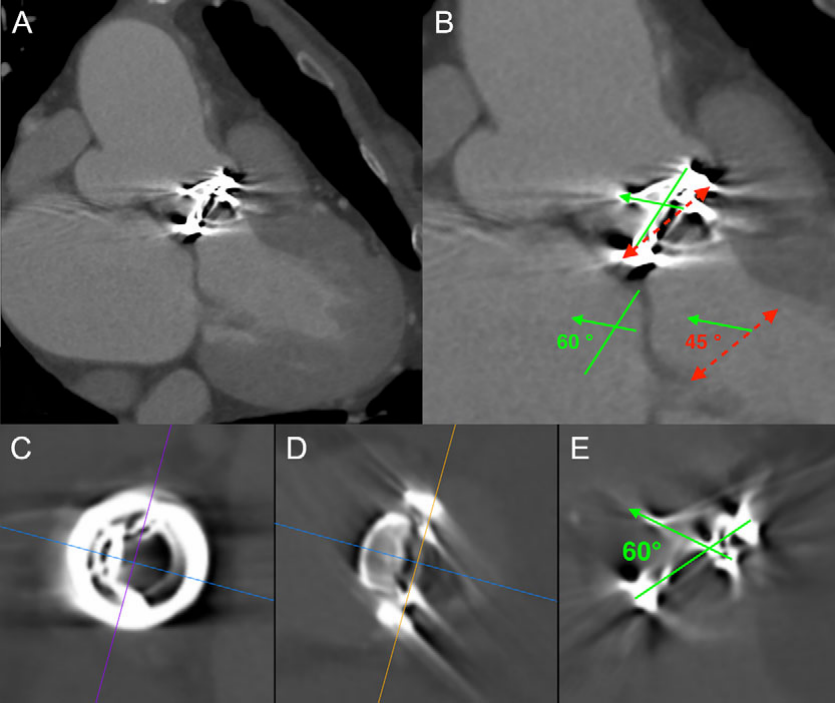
(A) Cardiac computed tomography (CT) angiography revealed a saccular ascending aortic aneurysm (maximum transaortic diameter: 51 mm) arising from the posterior ascending aortic wall just above the sino-tubular junction. (B) Cardiac CT angiography (systolic images) confirmed that the Björk-Shiley valve was tilted by the elevation of the prosthesis in the right-coronary sinus. Red dashed line: Aortic annulus (Basal ring), Green solid line: Prosthetic valve ring, Green solid arrow: Prosthetic valve leaflet. Physiologic opening angle calculated between the leaflet of the prosthetic aortic valve and the aortic annular plane was 45 degrees. (C, D, and E) Prosthetic heart valve assessment with cardiac CT (systolic images). Short axis view (C), frontal view (D), and lateral view (E) of the Björk-Shiley spherical-disc valve in the aortic position. Multiplanar reconstruction (MPR) image analysis revealed a normal leaflet opening angle of 60 degrees.
